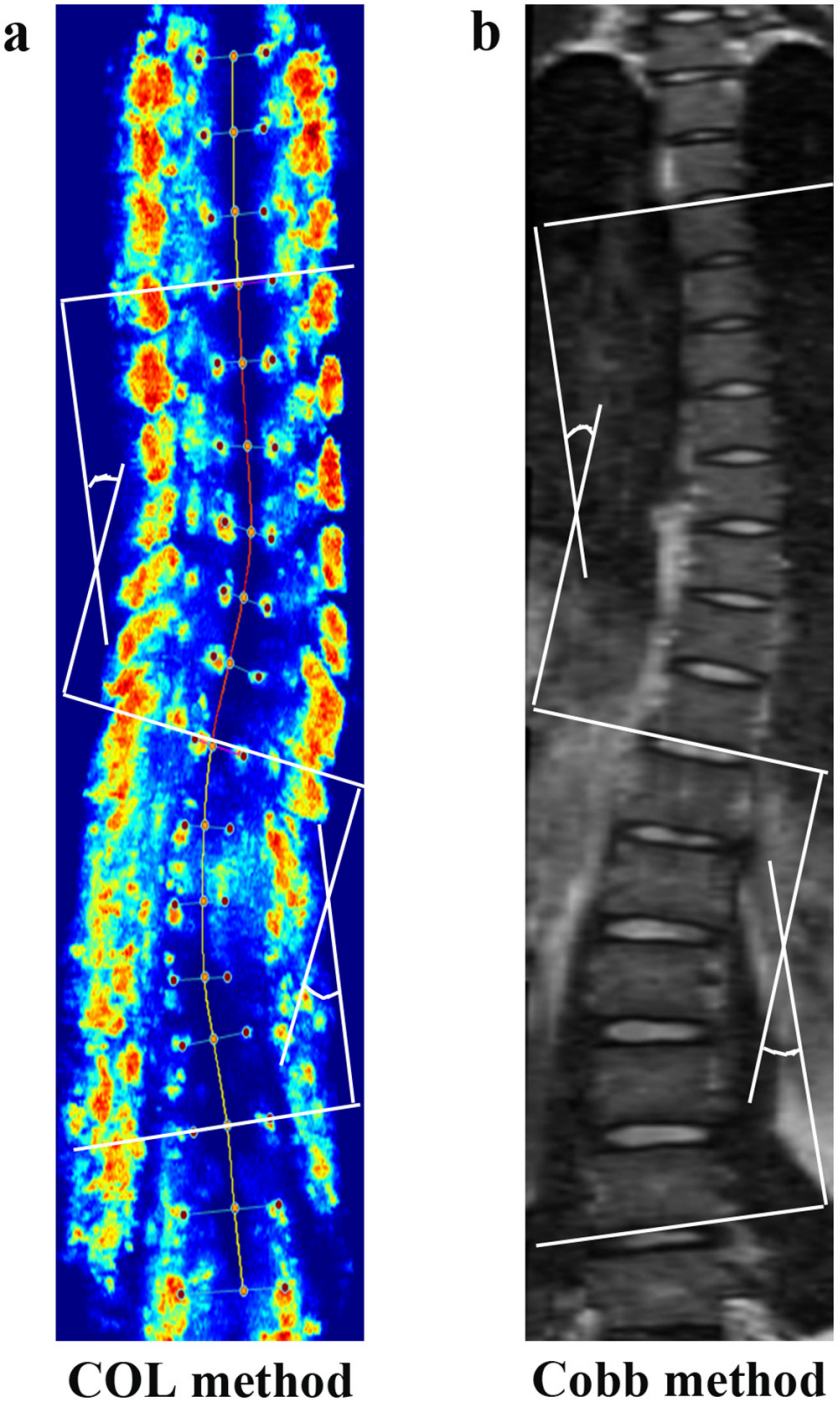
Spinal curvature measurement using. (a) Center of laminae (COL) method in ultrasound; (b) Cobb method in MRI.
